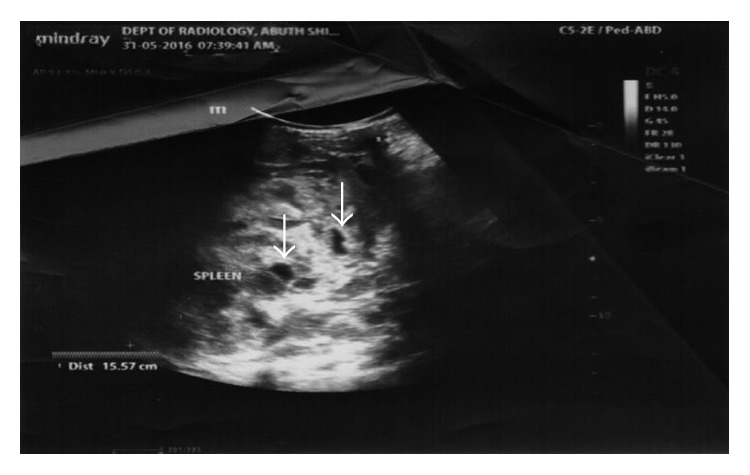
Abdominal ultrasound showing splenic metastasis.
